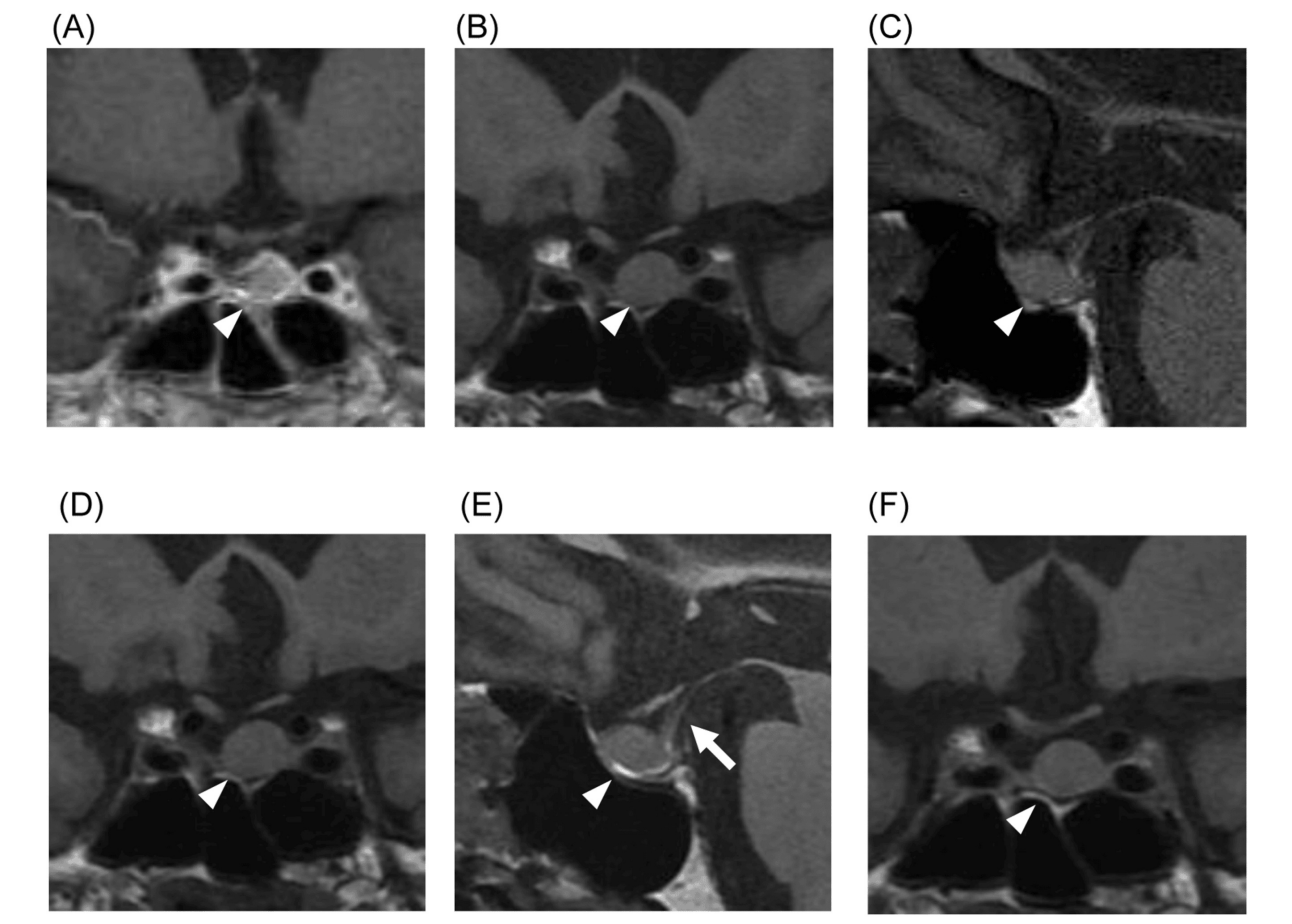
Pituitary T1-weighted MRI. White arrowheads indicate the pituitary gland. (A) Contrast-enhanced image taken 17 years before presentation, three months after surgery. (B) First admission, coronal section. (C) First admission, sagittal section. (D) Second admission, coronal section. (E) Second admission, sagittal section. Triangle marks indicate a pituitary adenoma, and the arrow indicates the pituitary stalk. (F) Contrast-enhanced image taken four months after diagnosis of adrenal insufficiency.
